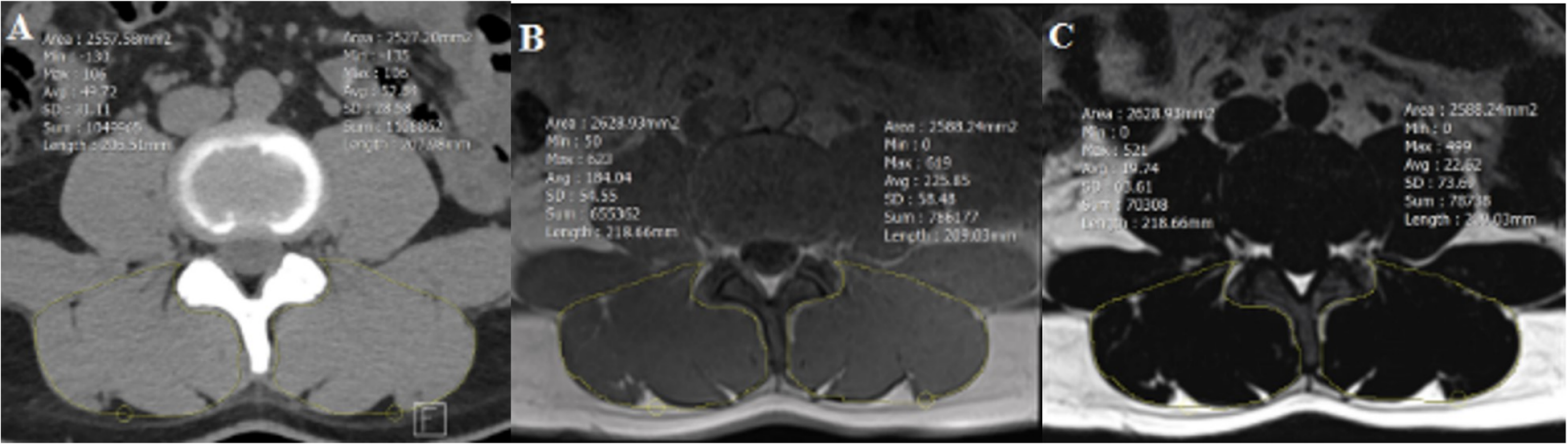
Image shows an example of the region of interest (ROI) of the paraspinal back muscle on axial plane and acquisition of the quantitative measurement values on PACS workstation. On CT (a), when the ROI is drawn, mean CSA (cross sectional area) and mean HU (Hounsfield unit) are displayed on screen. On MRI (b), when the ROI is drawn on in-phase image of two-point Dixon technique, the CSA and signal intensity values are displayed as on CT, and the ROI is copied and pasted onto the fat only image (c), then CSA and signal intensity measured
